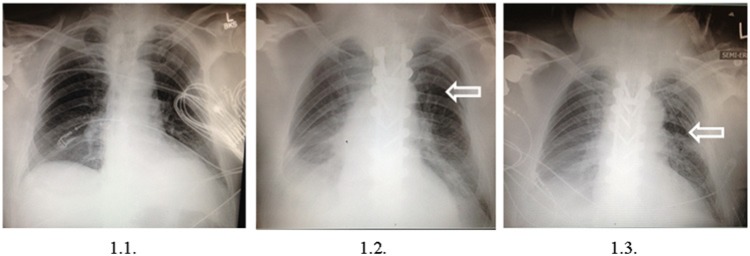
Results of the patient’s chest X-ray examinations. (1.1) Preoperative supine chest film. (1.2) Intraoperative supine film showing left pneumothorax. (1.3) Postoperative film showing left sided chest tube with improved lung markings.
